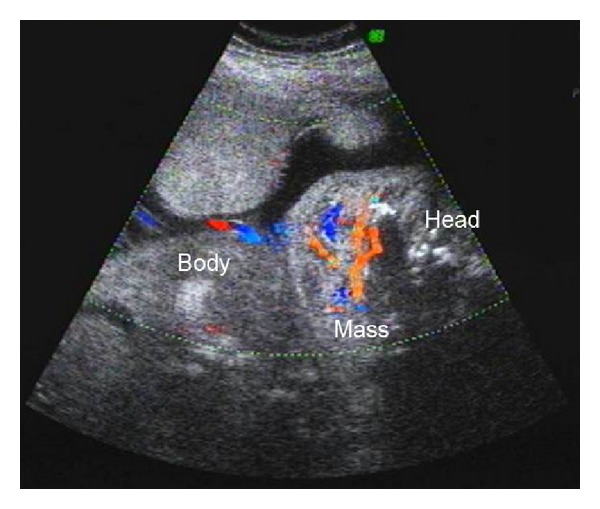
Ultrasound image at 29 weeks' gestation of a vascular neck mass—likely haemangioma.
